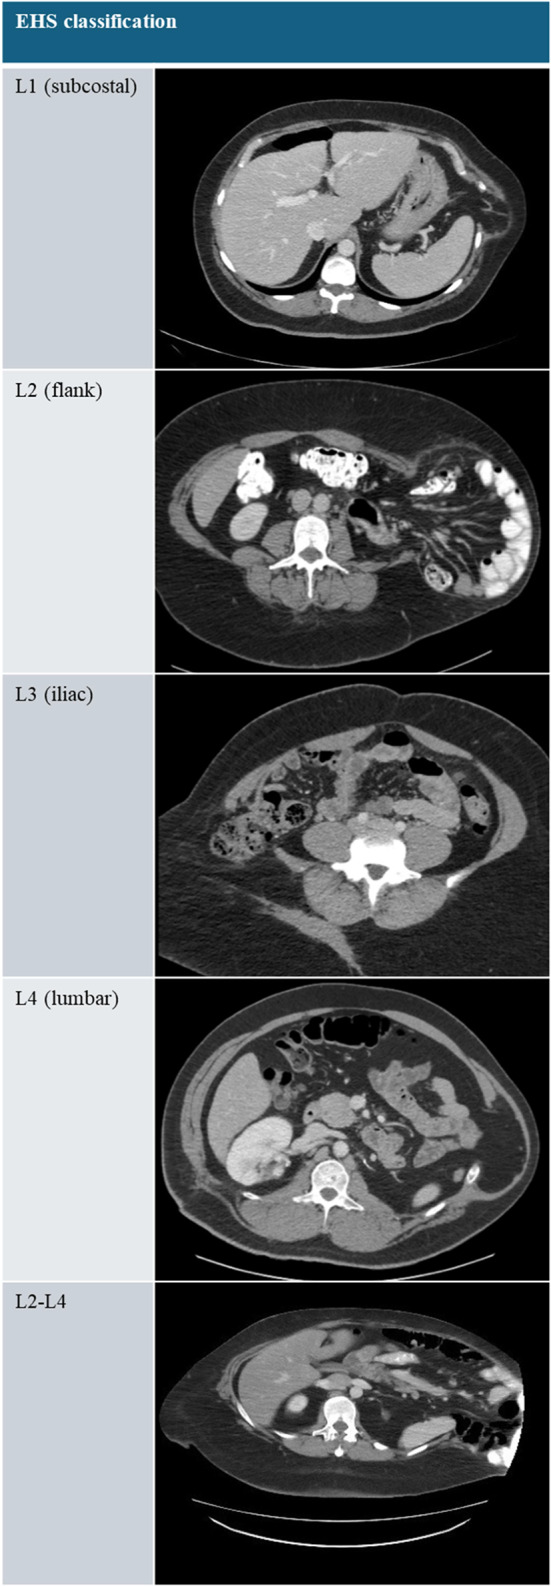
Representative CT images of European Hernia Society classification system for abdominal wall hernias
